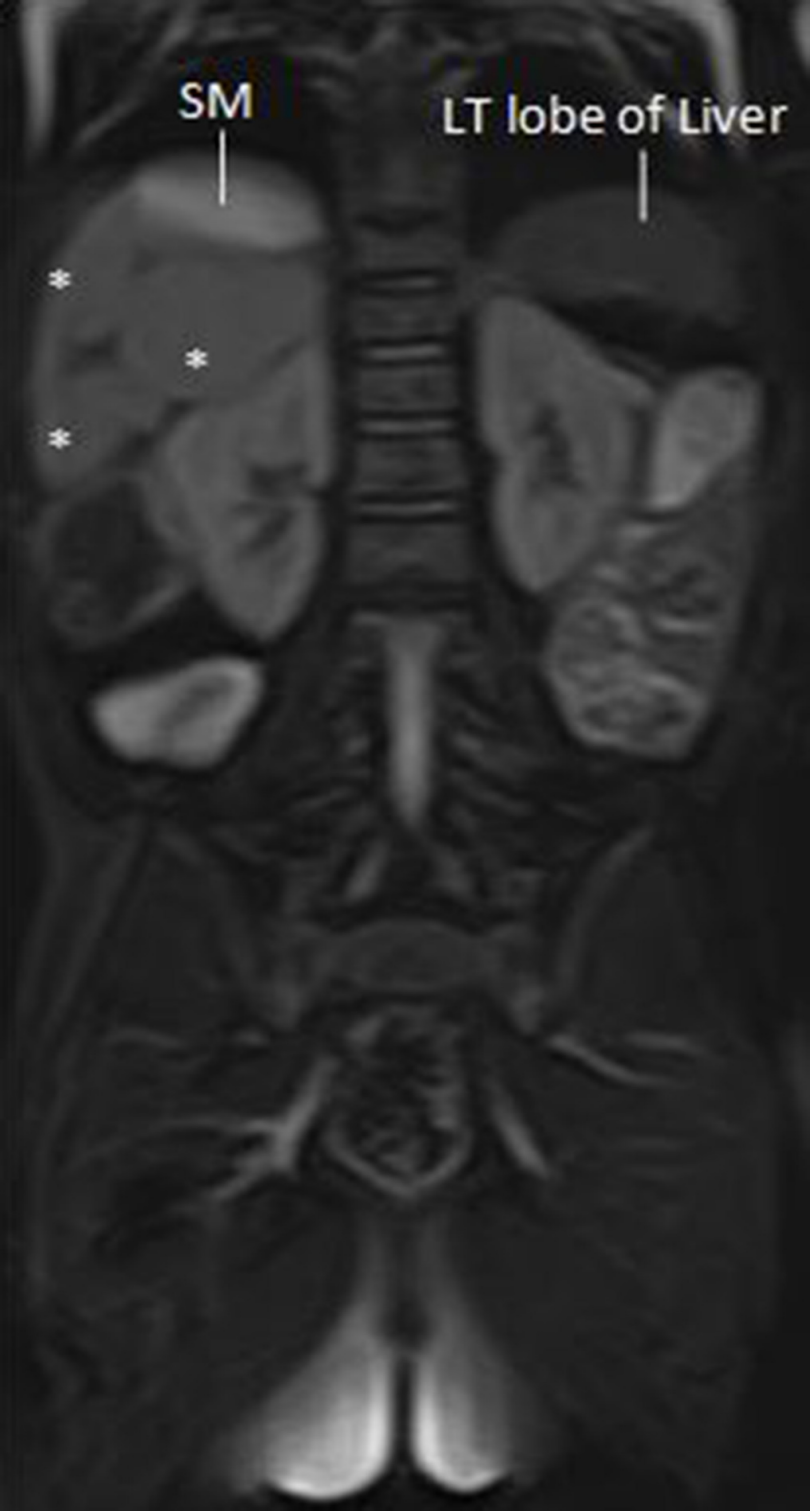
A *T*_2_ coronal MRI view showing multiple spleens (*) adjacent to the SM. SM, stomach; LT, Left.
